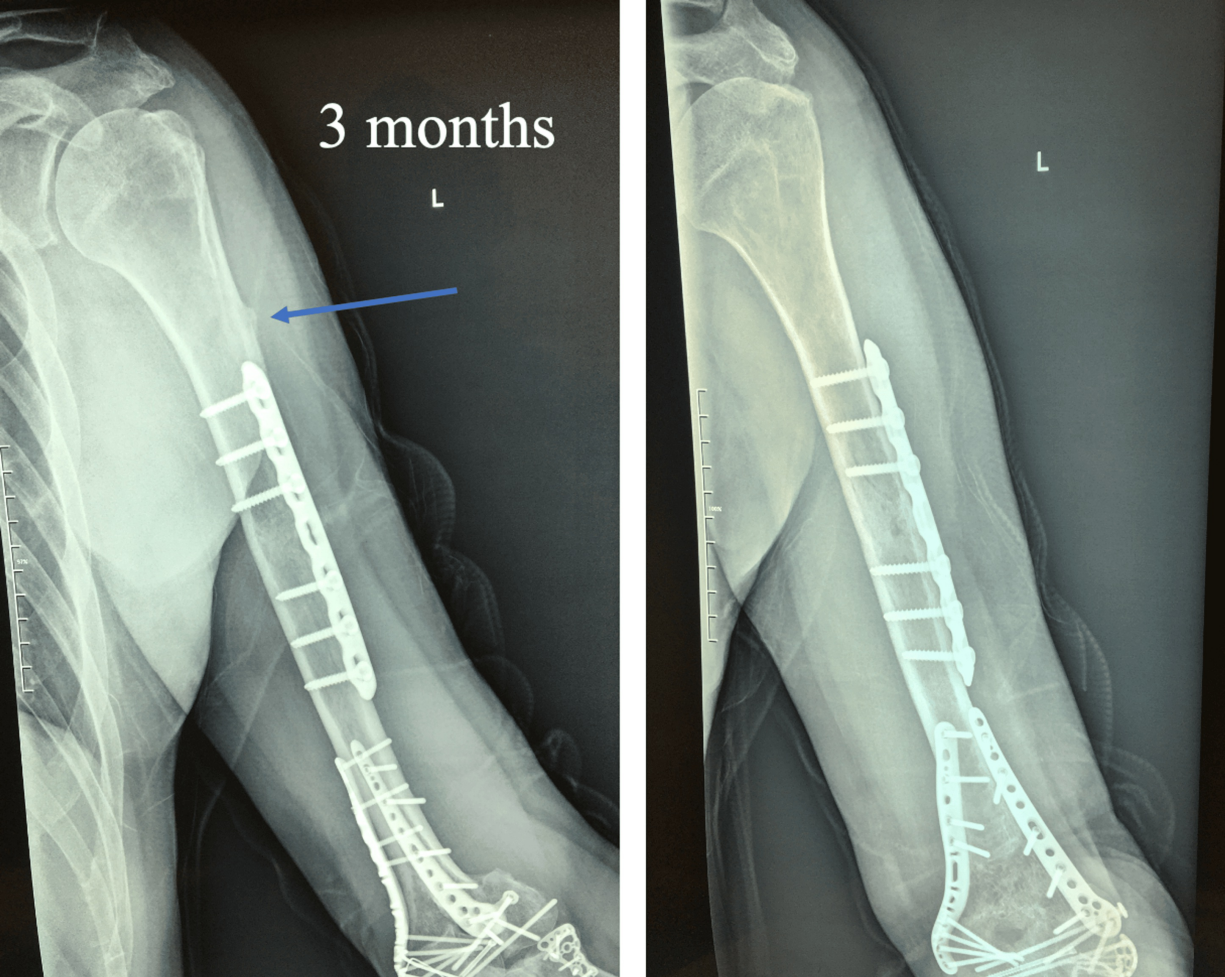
Radiographs at three months with the humerus in external rotation (left radiograph) and neutral position, in order to depict the fracture union. The union of the untreated proximal humerus is shown with the blue arrow.
